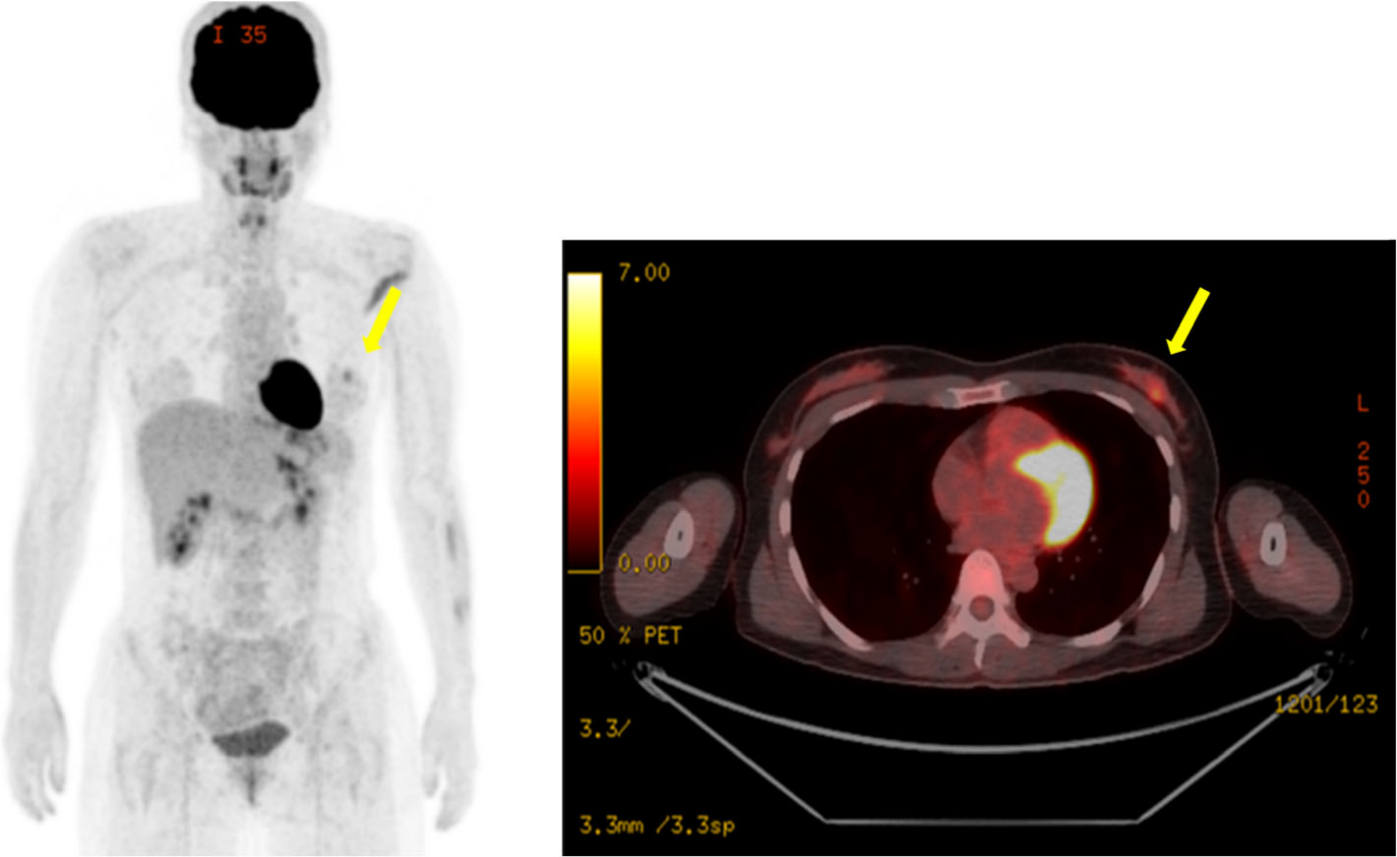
Whole-body fluorodeoxyglucose (FDG) positron emission tomography-computed tomography (PET-CT). Whole-body FDG PET-CT images showing a mass in the left C area with a maximum standardized uptake value of 3.54 (yellow arrows). Other abnormal accumulations were not detected in other axillary lymph nodes, lungs, liver, and bones
